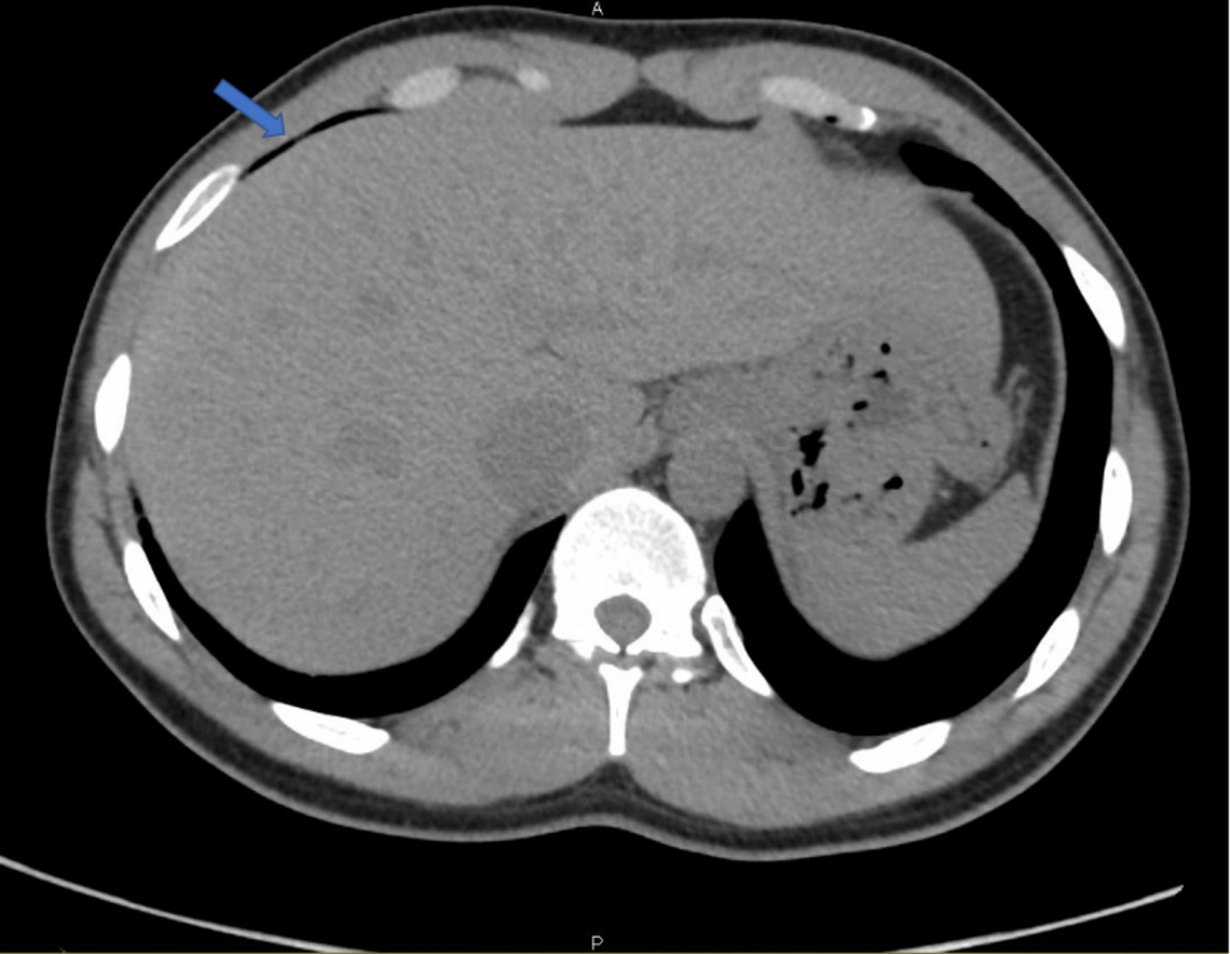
CT scan of the abdomen showing free air in the perihepatic region CT: computed tomography
